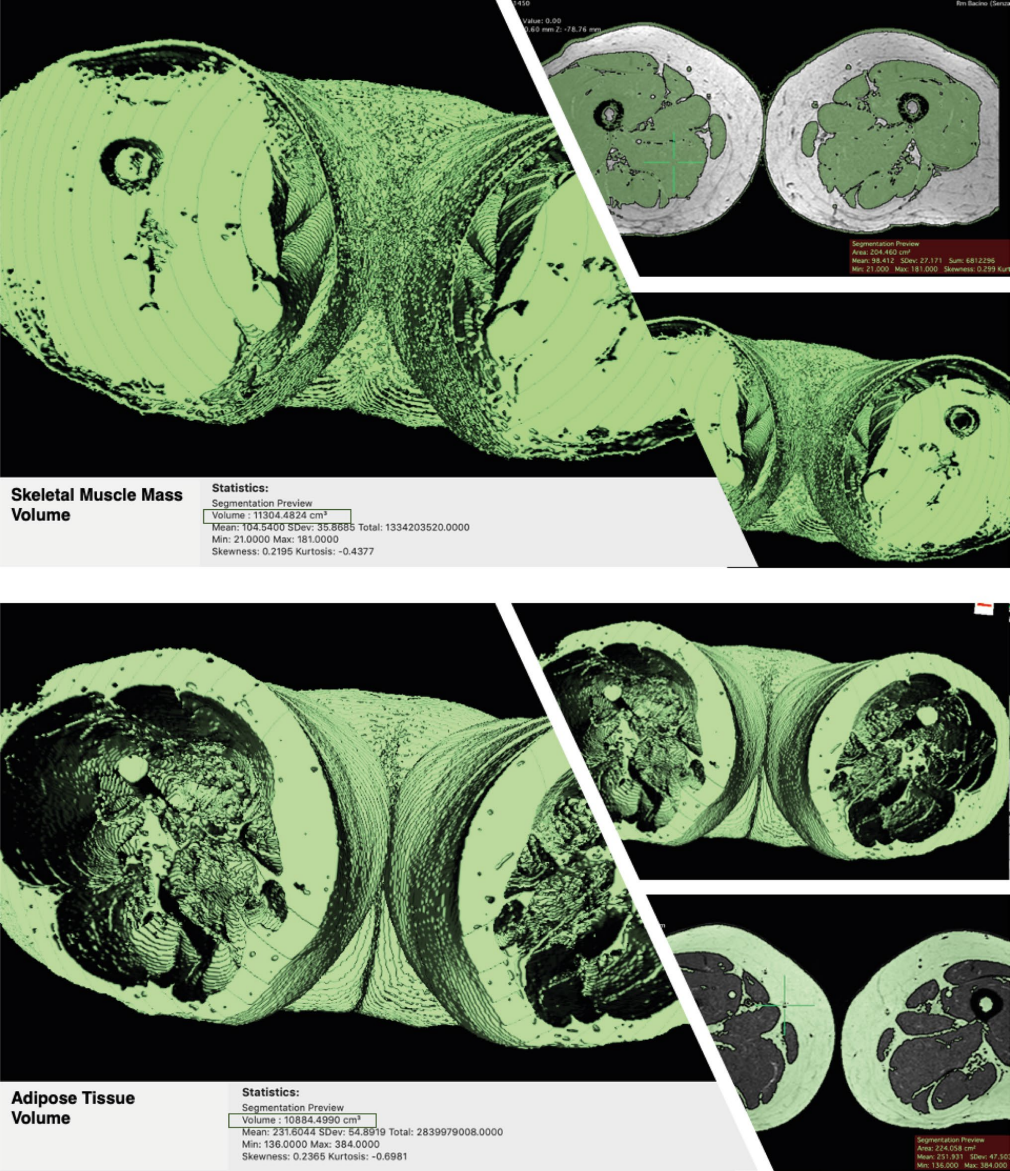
Skeletal muscle mass and adipose tissue volume reconstruction (software Horos) on MRI images. The quadriceps muscle volume reconstruction is based on the selection of the T2w dataset, selection of the command to generate the “Region of Interest,” insertion of the threshold interval, selection of the parameter “3D Growing region,” so that all the slices are considered, and application of the command “Brush ROI” to obtain a complete segmentation
